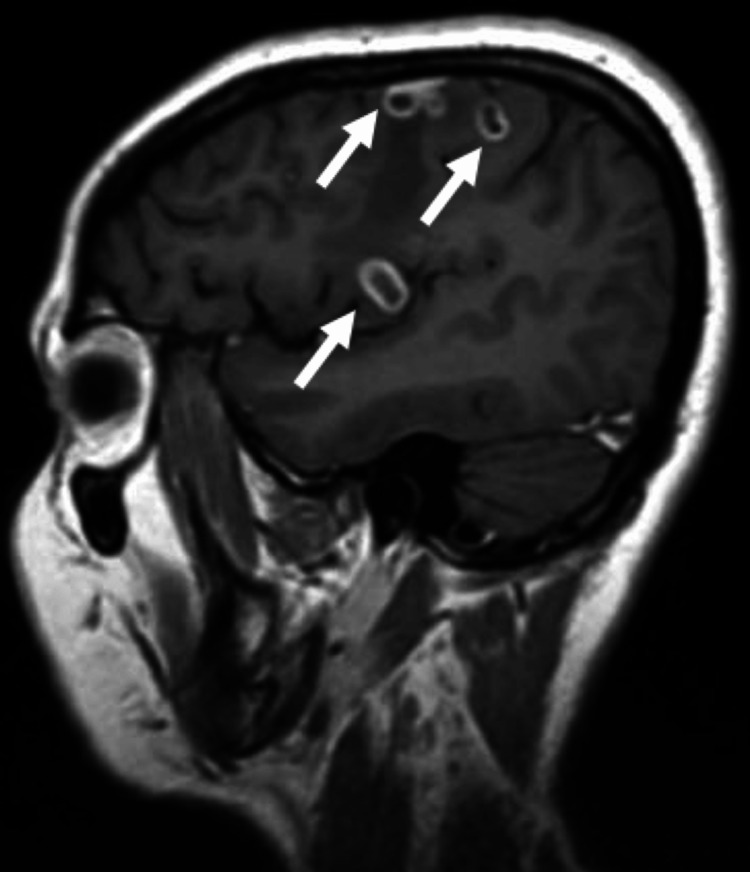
Contrast-enhanced with evidence of lesions suggestive of cerebral tuberculomas (T2-weighted imaging).
